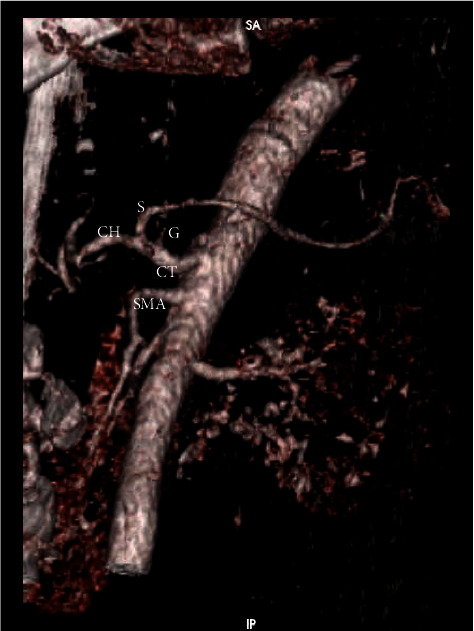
Three-dimensional volume rendering CT image shows normal branching patterns from the abdominal aorta. The celiac trunk (CT) is the most cephalad branch and gives off three branches: the splenic (S), left gastric (G), and common hepatic (CH) arteries. The superior mesenteric artery (SMA) arises at the L1 vertebral level from the aorta.
